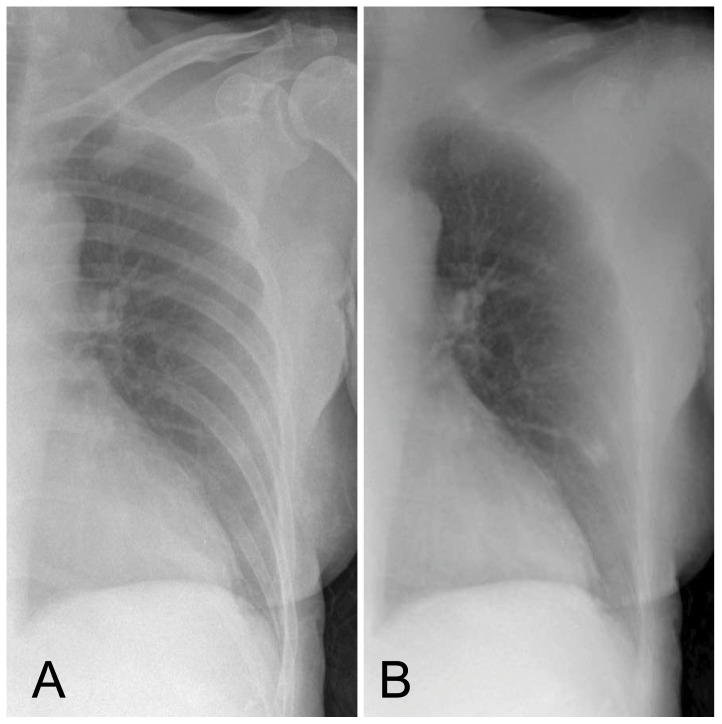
Example of a bedside case that was rated false positive by all 7 observers. Due to an incomplete suppression of the rib crossing of the anterior contour of the 5^th^ rib and the posterior contour of the 8^th^ rib on the BSI, the observers rated the shadow as infectious rounded opacity. Original radiograph (A), bone suppressed image (B).
